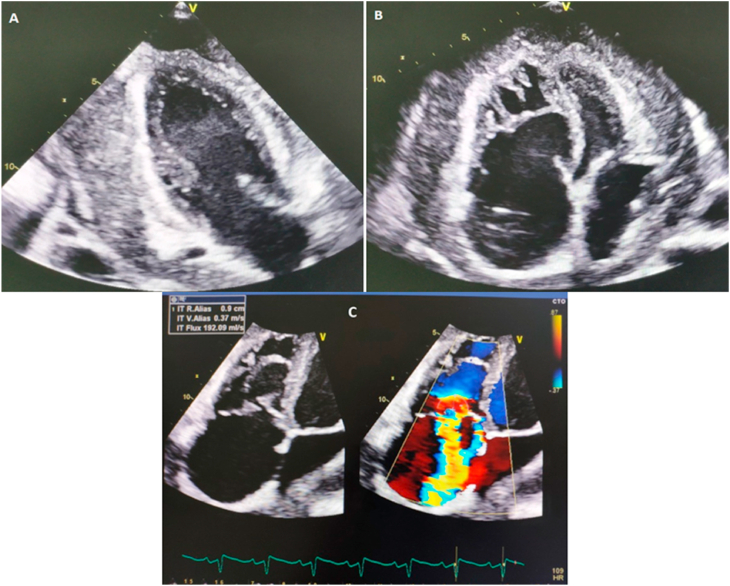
Transthoracic echocardiography showing a large circumferential pericardial effusion with significant fibrin deposits: (A) Apical 2-Chamber view, (B) Apical 4-Chamber view and (C) Severe tricuspid regurgitation.
